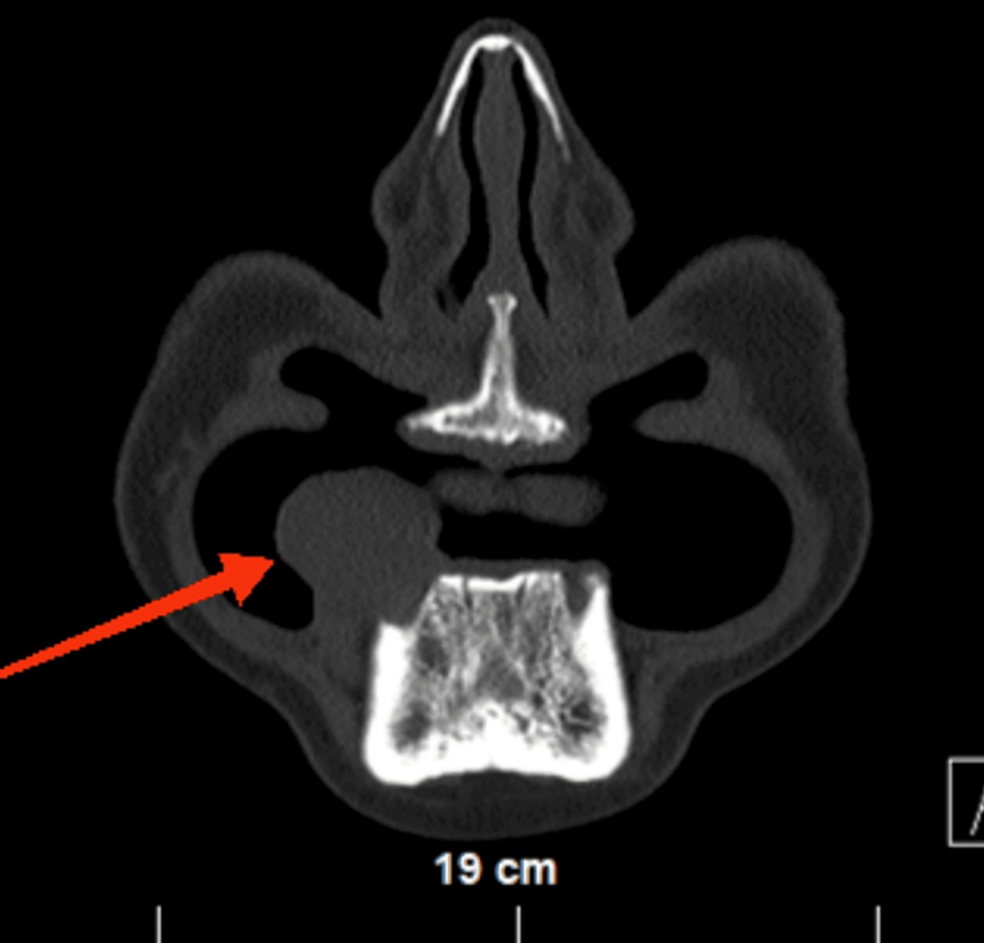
Axial section from a native CT scan of the head showing the tumor invasion in the alveolar process of the right mandible (red arrow)
